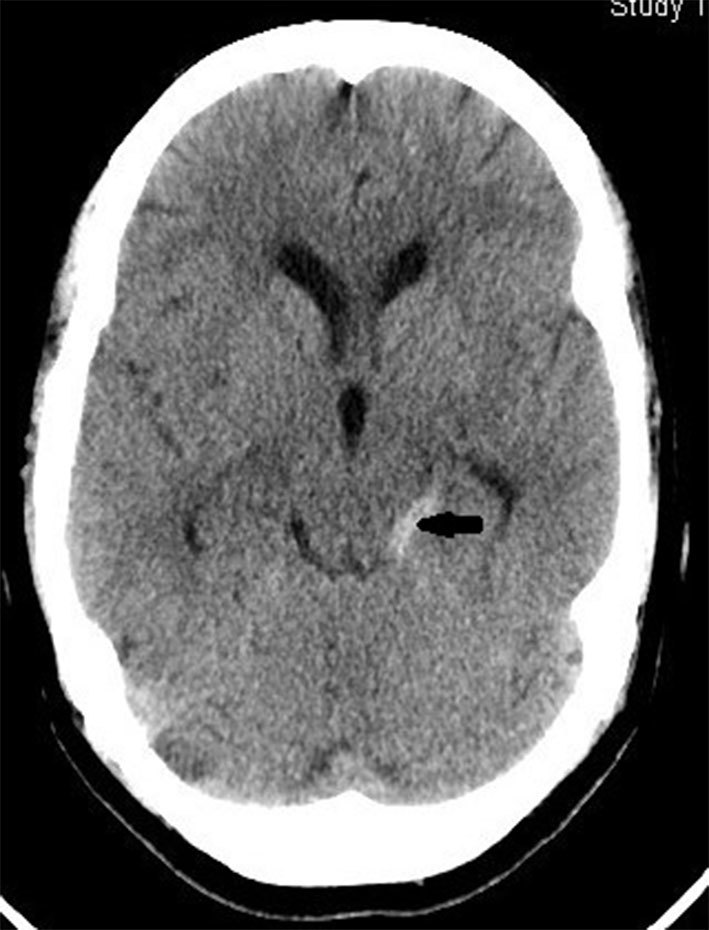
CT scan of the brain at the level of the mid-brain showing hyperdense blood in the perimesencephalic cistern on the left side (arrow).
